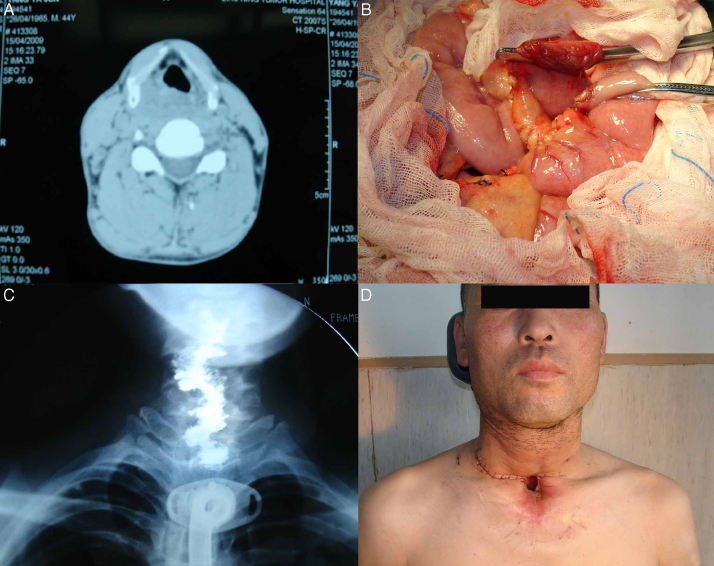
(A) Preoperative view of the hypopharyngeal cancer by CT; (B) the free jejunum flap was harvested from the left forearm; (C) barium swallow examination showing the postoperative result; (D) postoperative result 2 months after operation.
